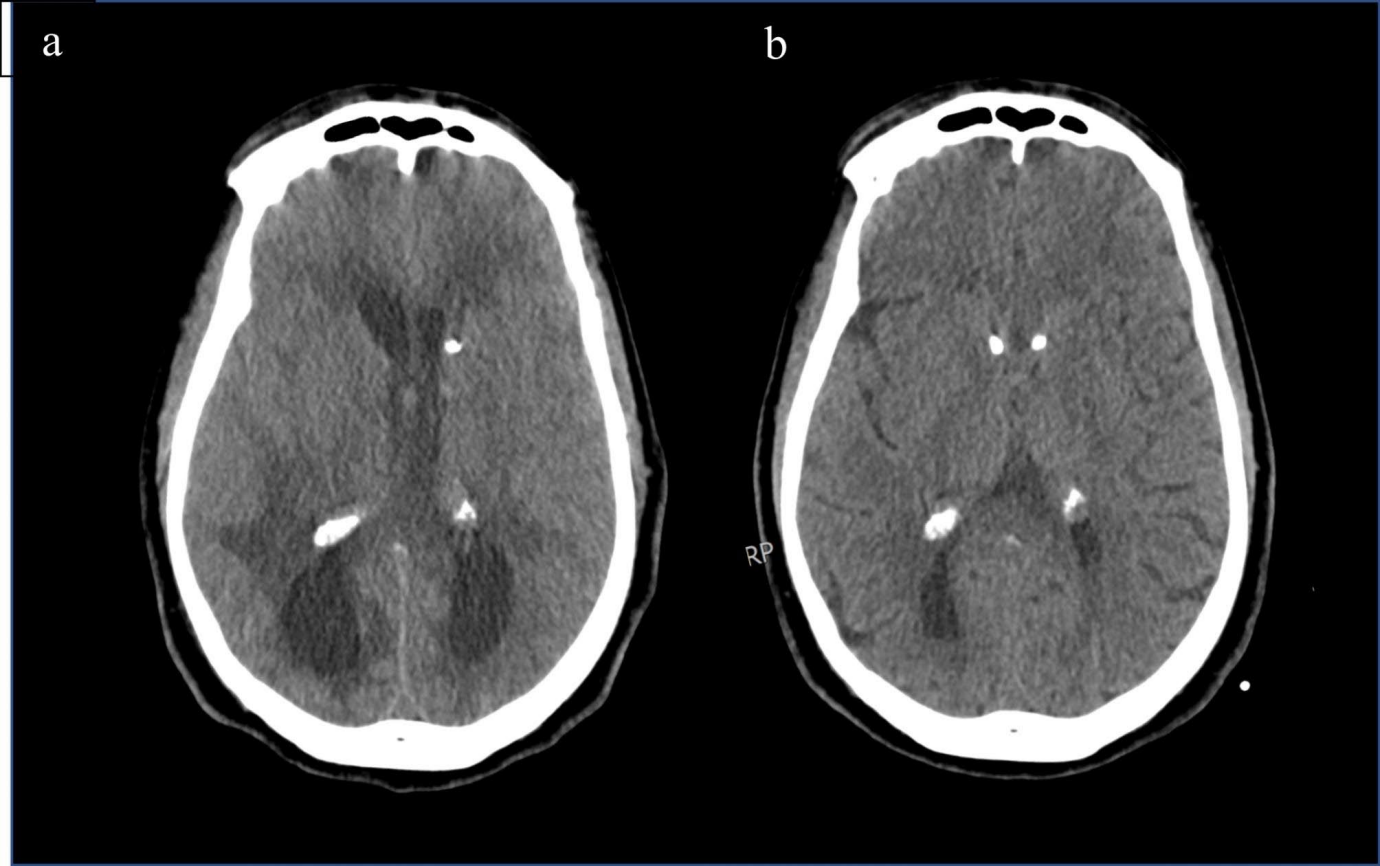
Non-contrast CT of the head after EVD obstruction demonstrating hydrocephalus and periventricular transependymal CSF seepage associated with sulcal effacement (a), with resolution of sulcal effacement after internalization of bilateral ventricular-peritoneal shunt drainage (b)
